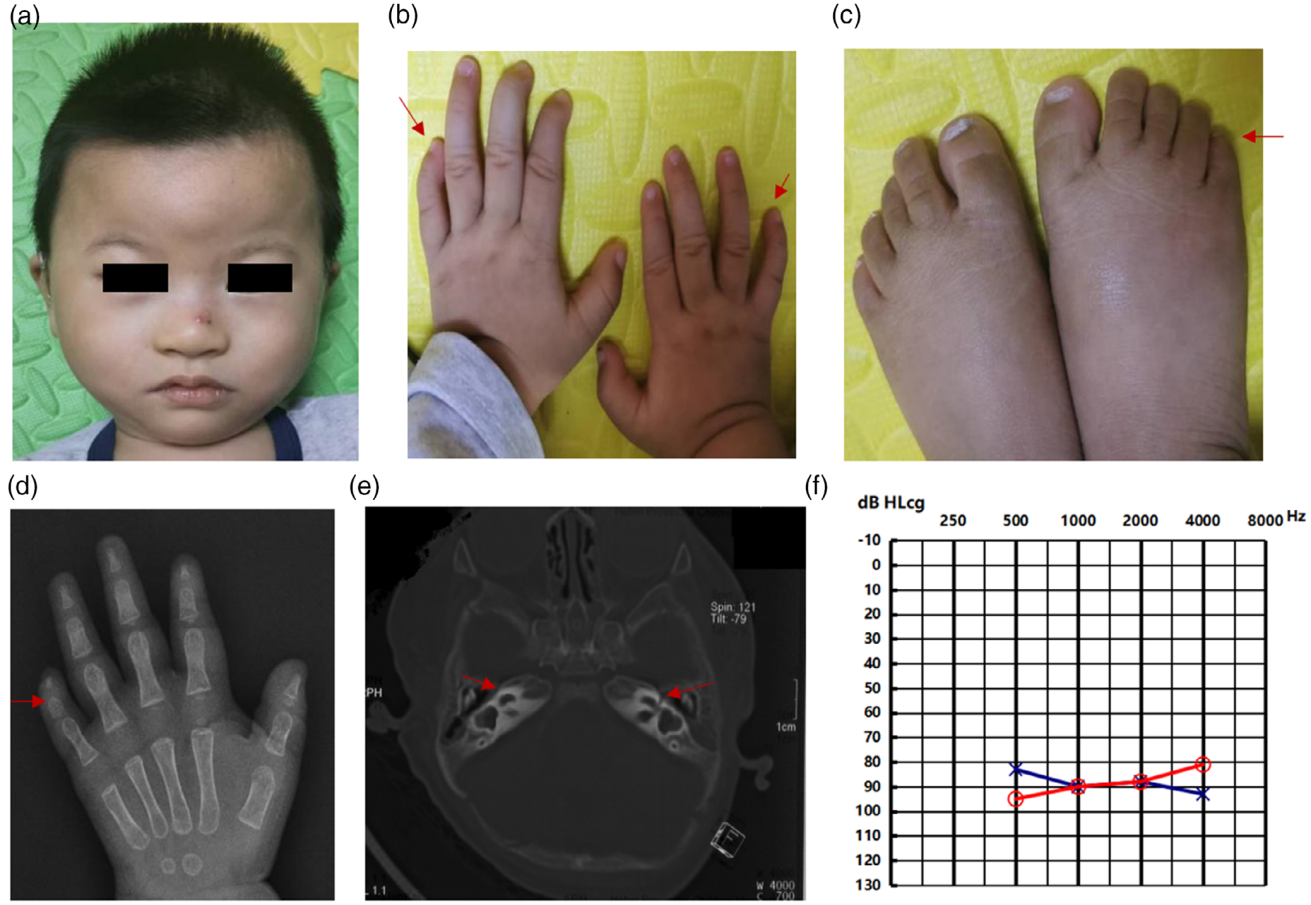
The phenotypic characteristics of proband 1. (a) The proband exhibited high anterior hairline, broad forehead with slightly flat zygomatic, low‐set ears with auricles that were turned backward, short nose with depressed nasal bridge, short columella with thick ala nasi, long and protruding philtrum, thin upper lip, and thick lower lip with teeth that were somewhat sparse. (b) The proband exhibited bilateral clinodactyly of the fifth finger with hypoplasia of the distal finger phalanx. (c) Feet demonstrating hypoplastic nails, with clinodactyly of the fifth toes. (d) X‐ray images of the left hand clearly showed hypoplastic distal phalanges of the fifth finger. (e) CT scans of the temporal bone revealed that the internal auditory canal was narrow on both sides of the inner ear, and the bilateral vestibular dilatation was cystic. (f) ASSR audiograms revealed showed severe to profound sensorineural hearing loss. Blue line—left ear, red line—right ear.
